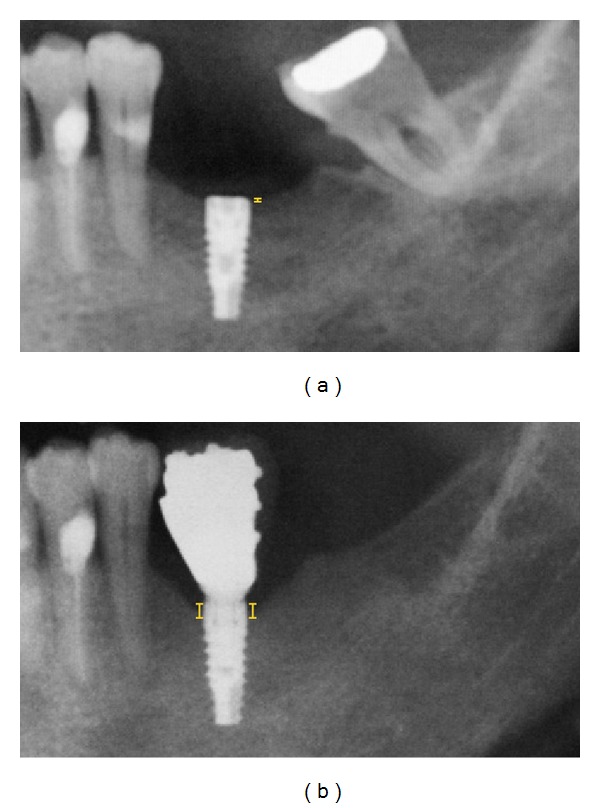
Detail of orthopantomographies 6 months (a) or 3 years (b) after implant insertion. MBL was calculated from the distance between implant shoulder and first bone-to-implant contact, which here is indicated by a yellow solid line.
